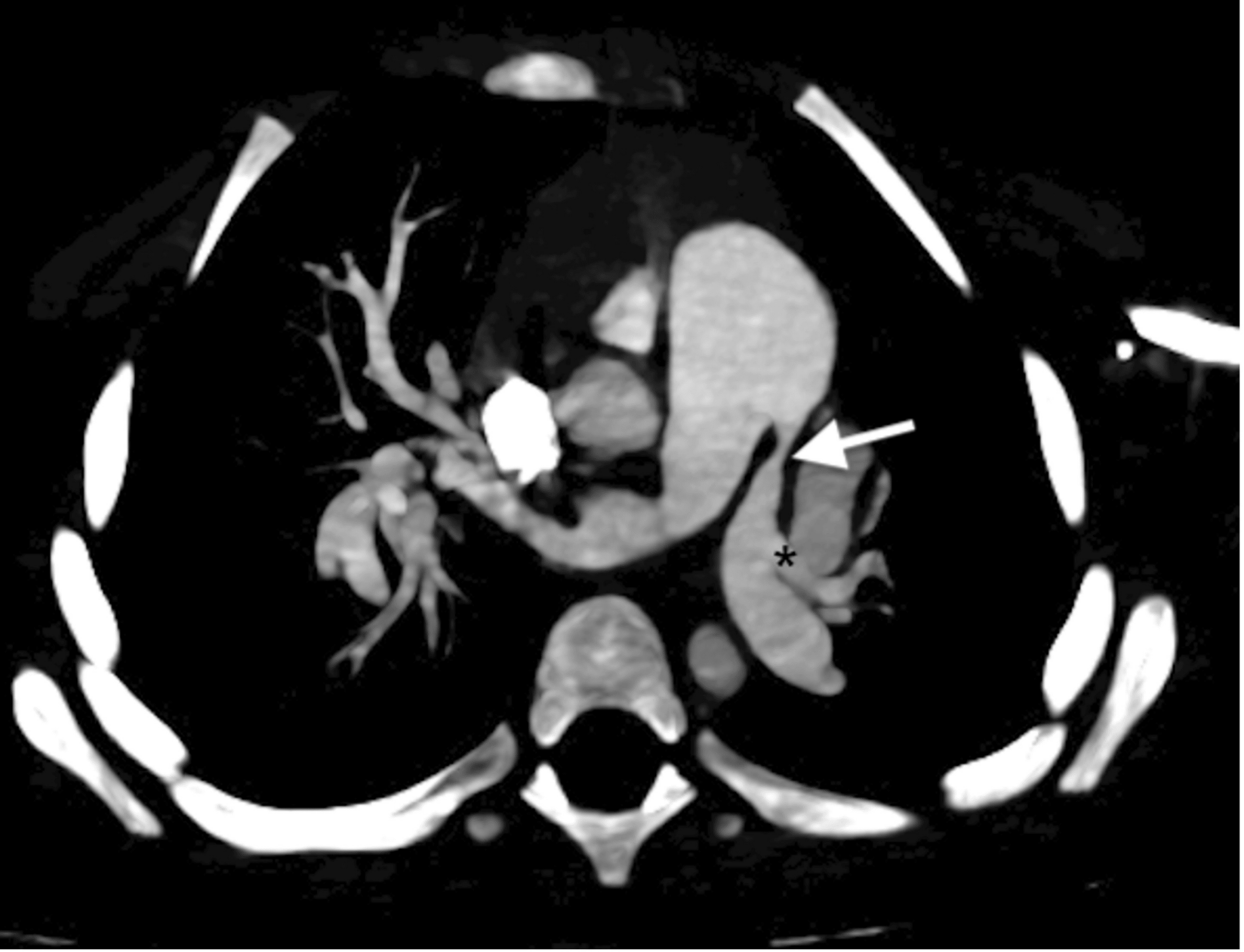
Maximum intensity projection. The CT image shows stenosis of the left main pulmonary artery at its origin (white arrow) and its abnormal second branch (asterisk).
